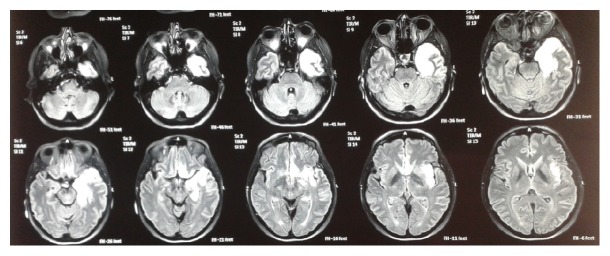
MRI brain scan. Notice the hyperintense depiction of the left temporal and the frontal lobe and the island of Reil in T2WI and FLAIR sequence.
